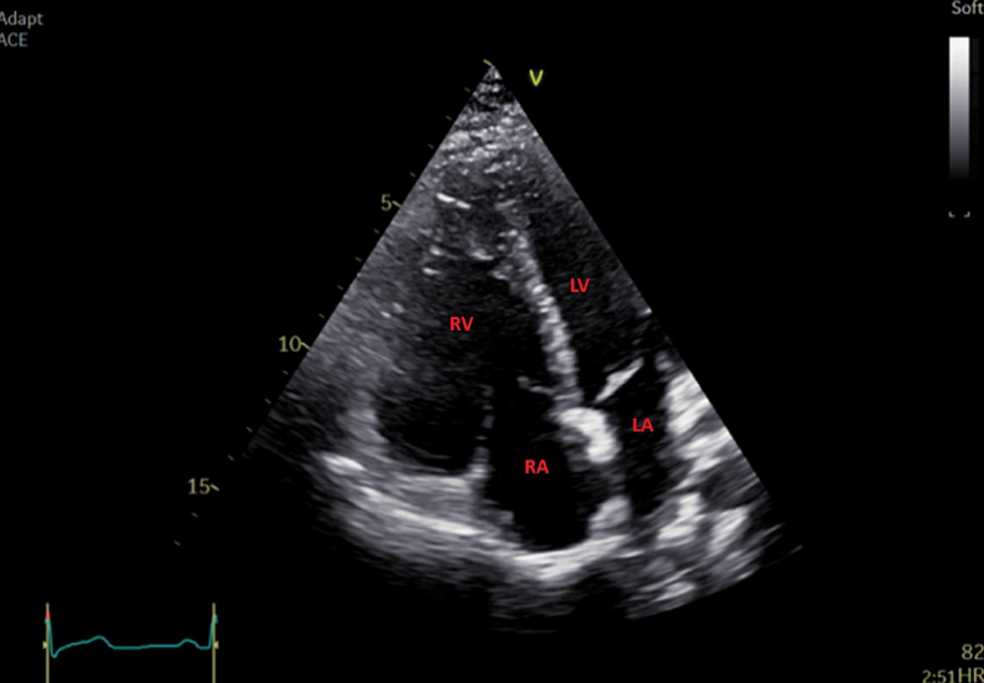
Modified apical four-chamber view on TTE showing dilated right ventricle. RV: right ventricle; RA: right atrium; LV: left ventricle; LA: left atrium; TTE: transthoracic echocardiogram
